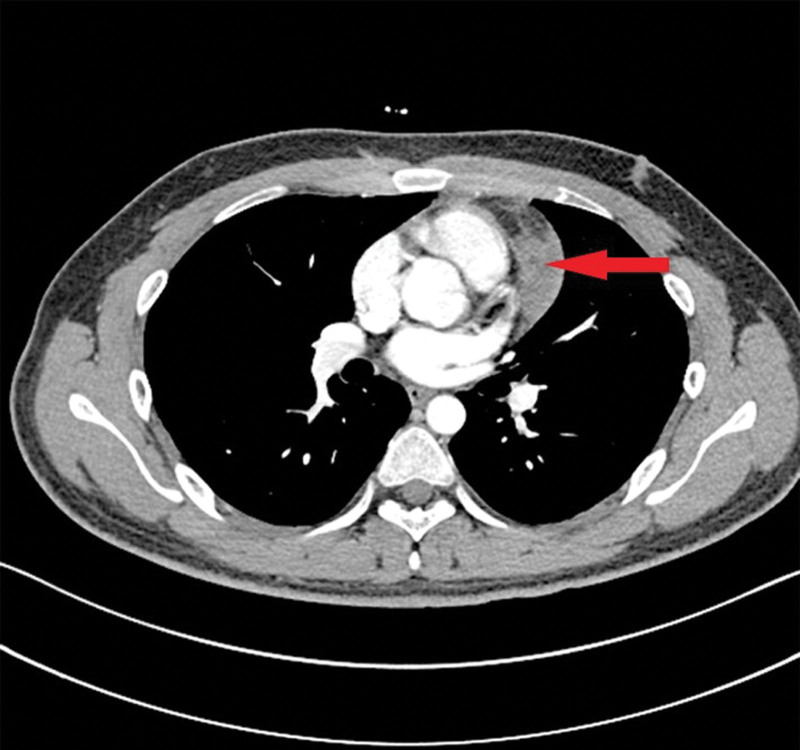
Imaging: Chest enhanced CT showed a mass of mixed density shadow on the left side of the anterior mediastinum. CT = computed tomography.
